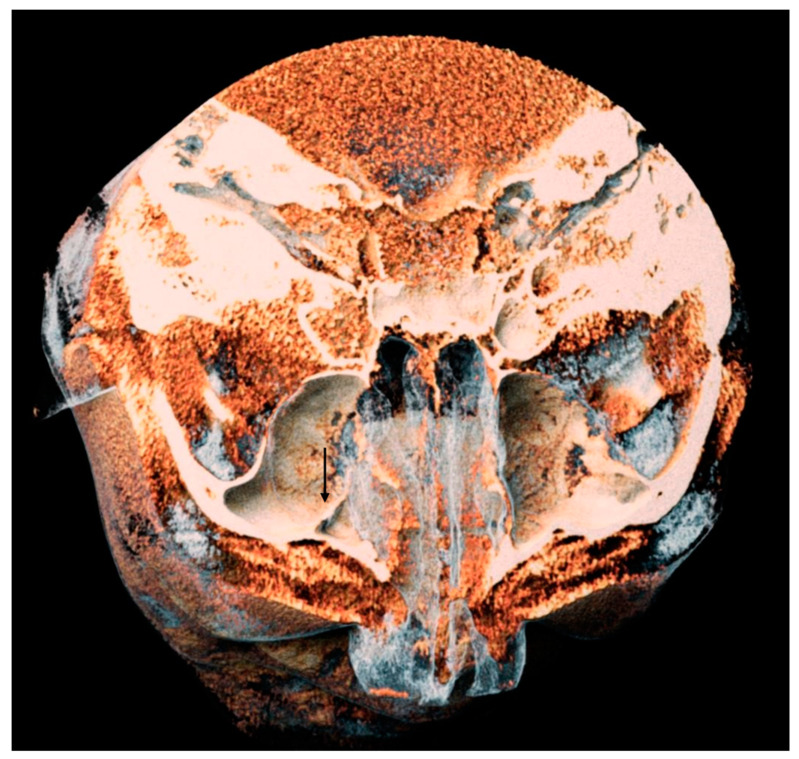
A 3D volume rendering of an analyzed CBCT. Image obtained from Anatomage^®^. It is possible to appreciate on the left side a septum of the maxillary sinus, indicated by the black arrow.
